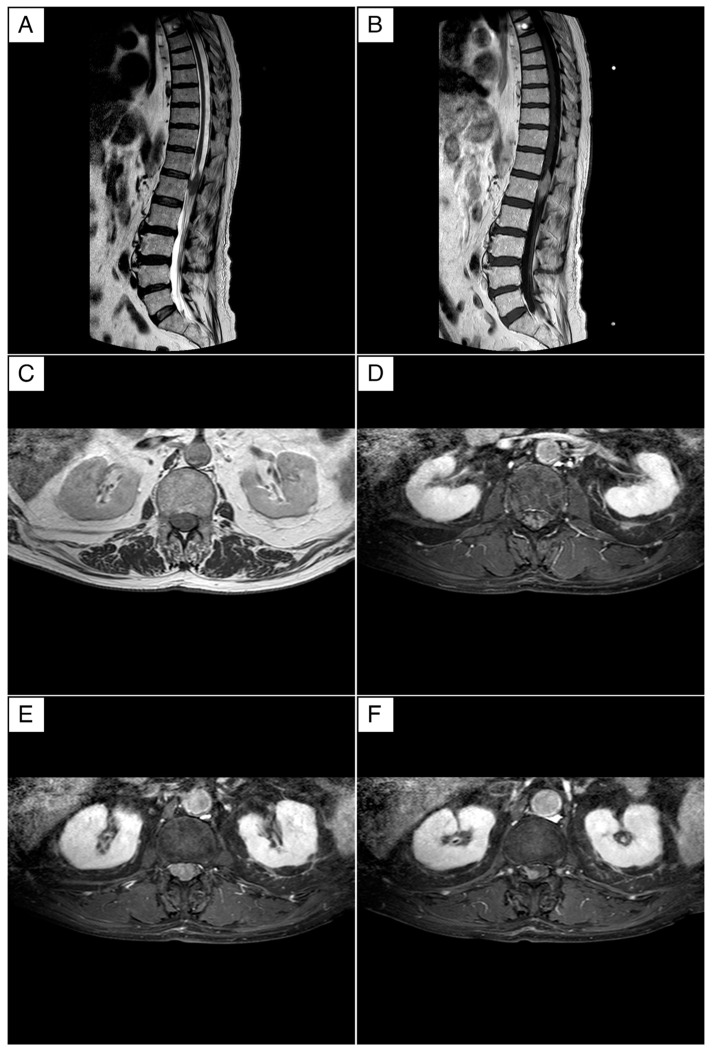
Magnetic resonance imaging (MRI) of the dorso–lumbar spine. (A) The T2-weighted sagittal MRI demonstrates an extra-axial lesion exhibiting a hypointense signal. (B) The T1-weighted sagittal MRI demonstrates a lesion exhibiting a hyperintense signal. (C) The T2-weighted axial MRI demonstrates a structure that compresses but appears to not infiltrate the cauda. (D–F) Gadolinium-enhanced T1-weighted axial MRI shows an extra-axial lesion with homogeneous signal enhancement. This lesion appears to be of extra axial origin, and the characteristics of signal and enhancement can be attributed in the initial hypothesis to a meningioma with a probable short right anterolateral implant in accordance with an image attributed to a dural tail.
